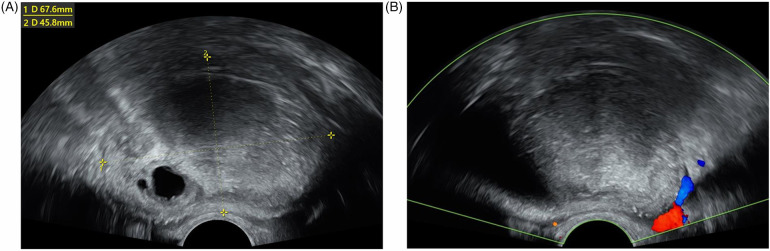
Ultrasound images from a 17-year-old girl with a confirmed mature teratoma. (A) Conventional ultrasound image shows a classic benign lesion with a diameter of 6.8 cm (calibers). (B) Color Doppler image shows no vascularity (Color score = 1). The mass was classified as O-RADS 2.
